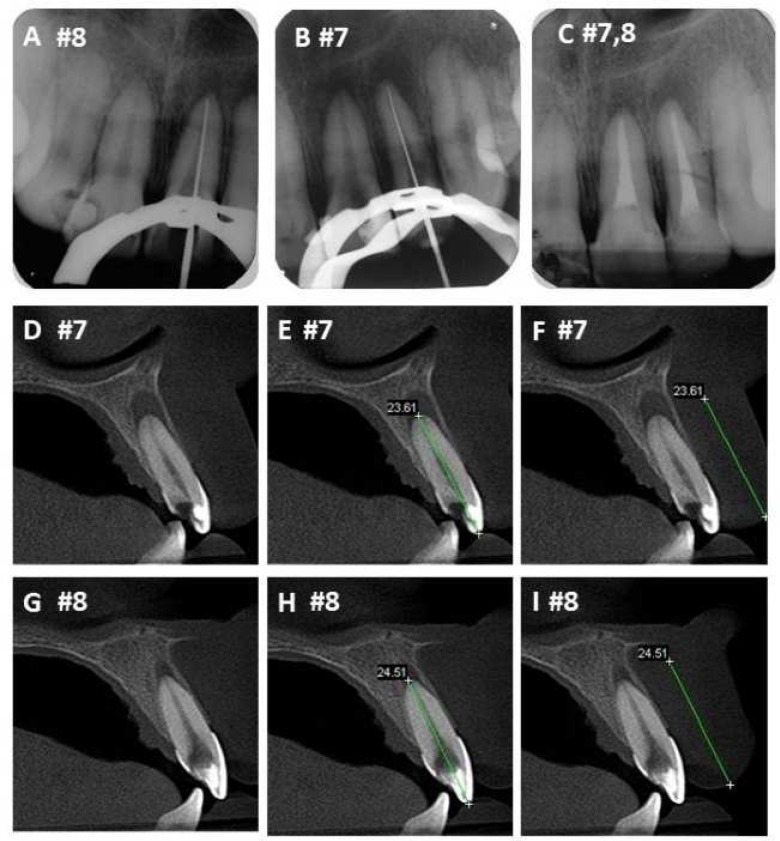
Determination of working length in maxillary central and lateral incisors: *A to C)* periapical radiographies and *D to I)* CBCT images
